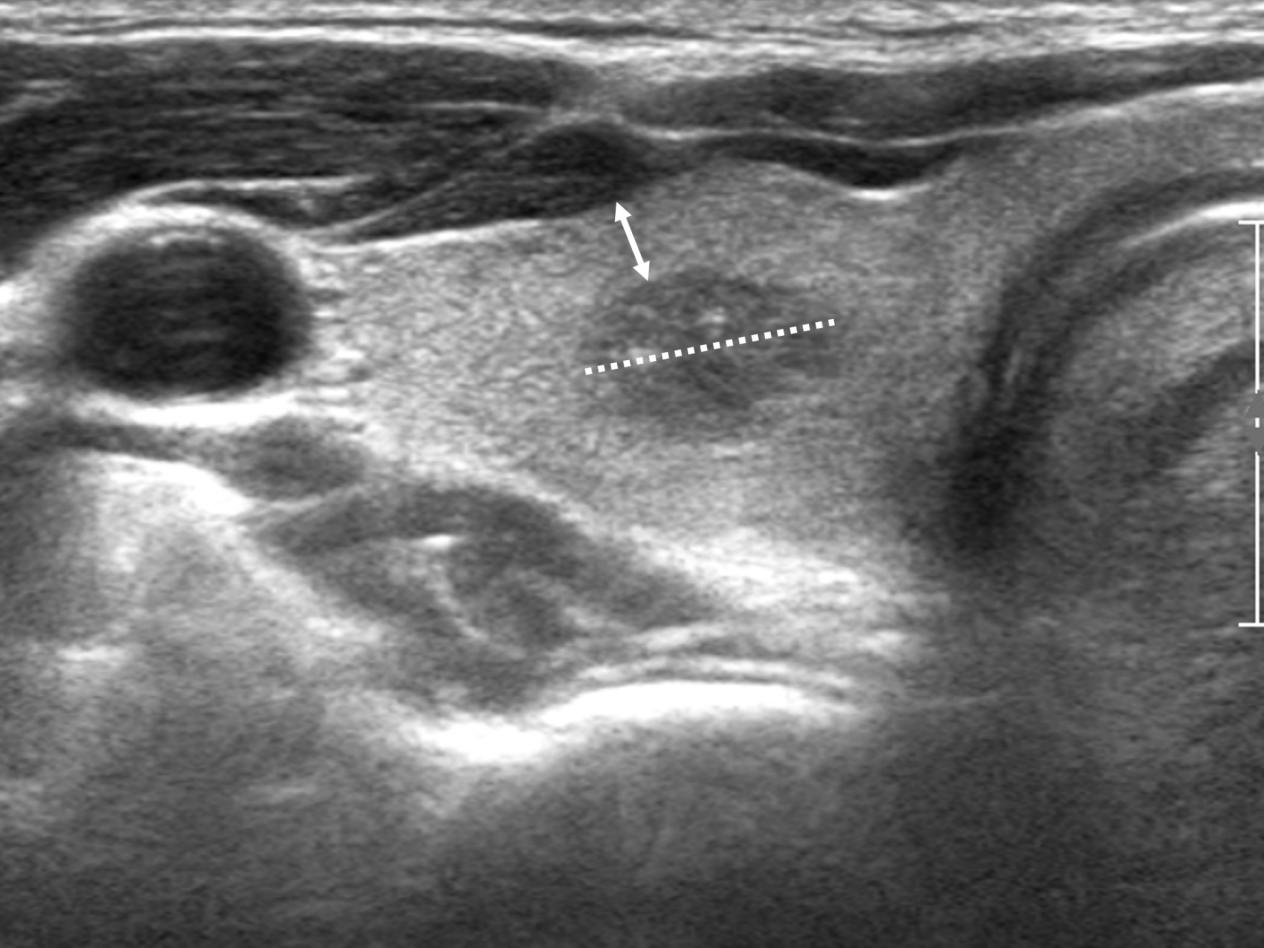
Tumor size and distance from capsule. Measurement of tumor size (dotted line) and distance from capsule (double headed arrow) on ultrasonography images.
